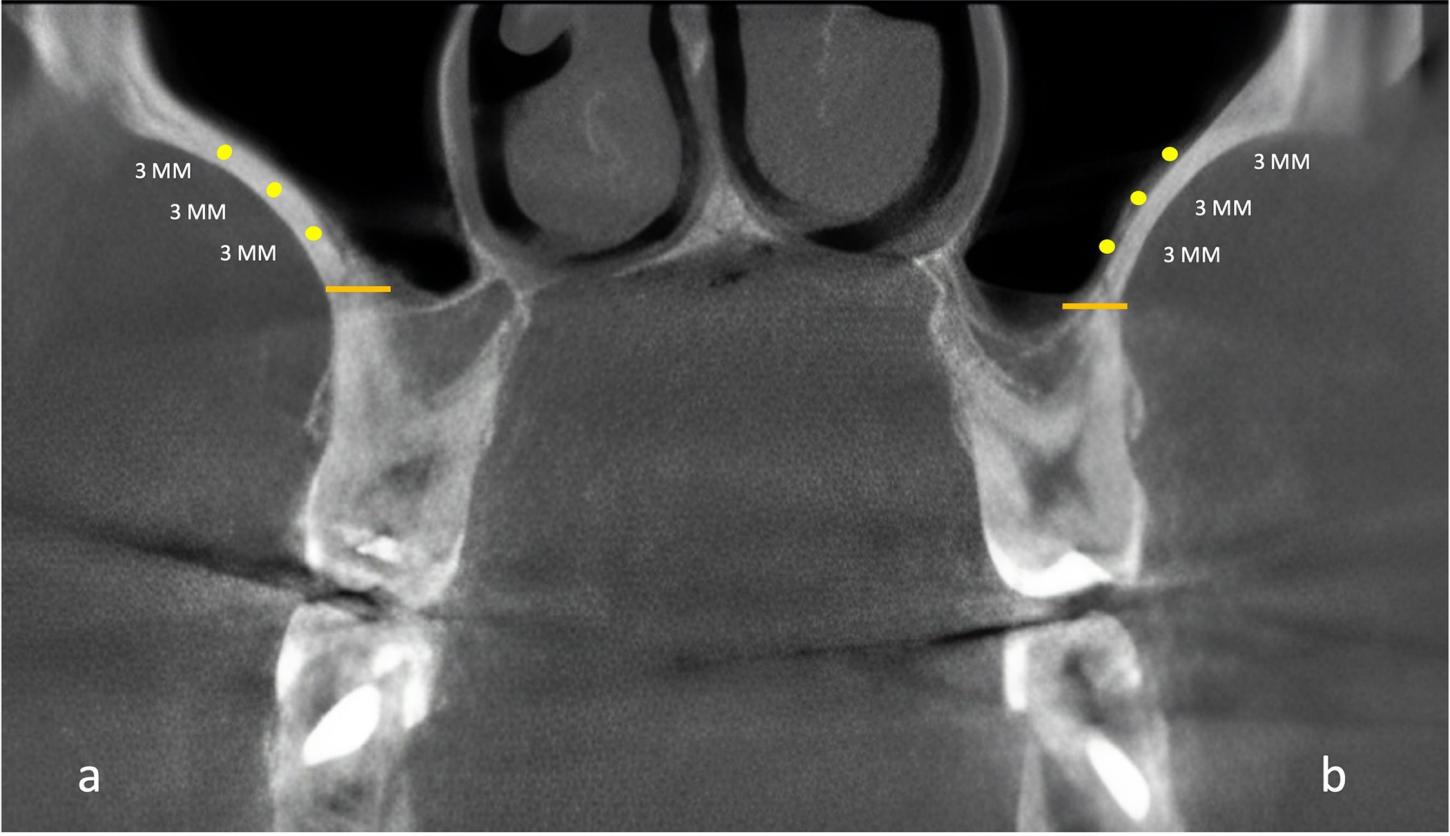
Coronal section of CT scan at the level of maxillary sinus. (A) reference point on lateral wall at 3mm distance form base of the sinus floor, (B) reference point on sinus membrane at 3mm distance from base of the sinus floor).
